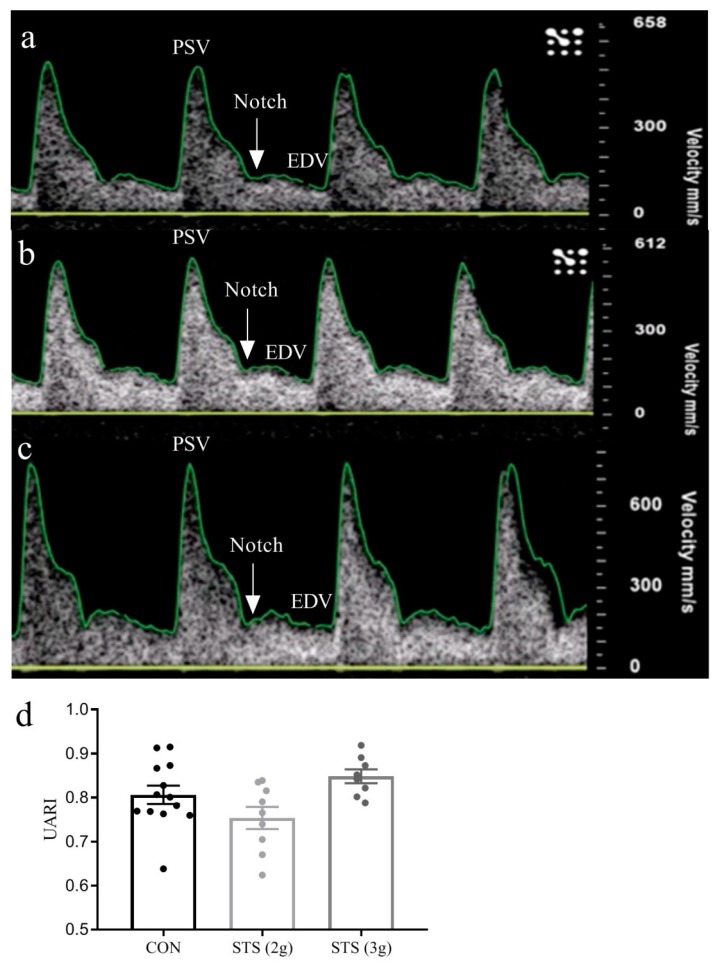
Uterine artery resistance index on GD18. Examples of Doppler waveforms in a (a) control dam; (b) dam treated with 2 g STS per kg body weight per day; and (c) dam treated with 3 g STS per kg body weight per day, white arrows indicate notching; (d) treatment with 2 g STS (n = 9) and 3 g STS (n = 8) showed no significant difference in UARI as compared with the control (n = 13) with persistence of characteristic notching tested with one-way ANOVA Dunnett’s multiple comparisons test, data presented as mean ± S.E.M. CON, control; EDV, end-diastolic flow velocity; GD, gestational day; PSV, peak systolic flow velocity; STS, sodium thiosulfate; UARI, uterine artery resistance index; 2 g, 2 g per kg body weight per day; and 3 g, 3 g per kg body weight per day.
